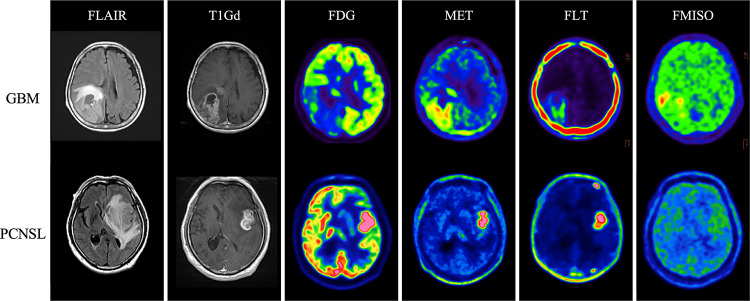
Representative cases of GBM and PCNSL with PET tracer uptake values. Upper panel: （Case1) a 71-year-old female with GBM. PET tracer uptake values (TNR and TBR): FDG 1.13, MET 4.60, FLT 8.43, FMISO (TBR) 3.05. Lower panel: (Case2) a 77-year-old male with PCNSL. PET tracer uptake values (TNR and TBR): FDG 2.59, MET 7.75, FLT14.68, FMISO (TBR) 1.45. FDG, [^18^F]fluoro-2-deoxy-D-glucose; MET, L[methyl[^11^C]]methionine; FLT, 3′deoxy3′[^18^F]fluorothymidine; FMISO, [^18^F]fluoromisonidazole; PCNSL, primary central nervous system lymphoma; TBR; tumor-to-blood ratio; TNR, tumor-to-normal ratio; GBM, glioblastoma multiforme
